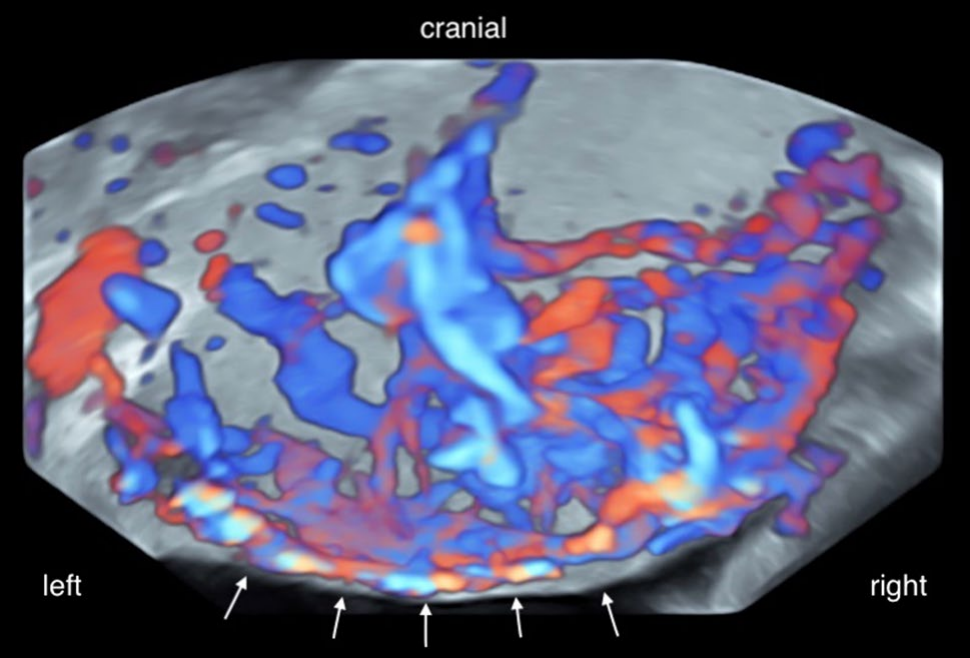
Transvaginal sonography in 22 + 6 weeks of gestation, colour mode, glassbody mode. Pattern of vascularisation at the interface between placenta and bladder wall with bridging vessels. Bladder wall is marked with arrows
